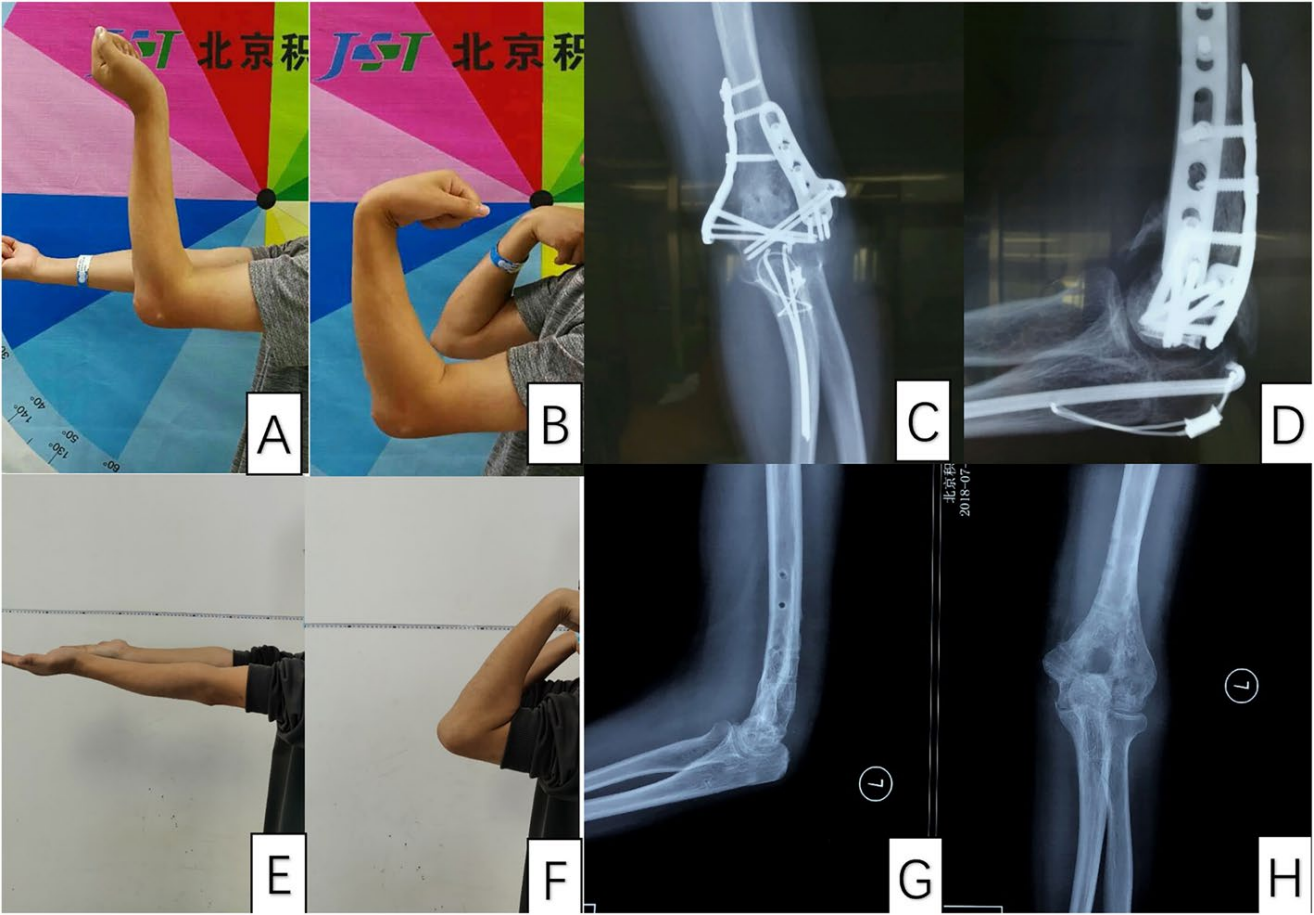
Elbow function and X-ray preoperatively and postoperatively. (A and B) Preoperative extension and flexion. (C and D) Preoperative X-rays. (E and F) Extension and flexion at the last follow-up. (G and H) The X-rays at the last follow-up
